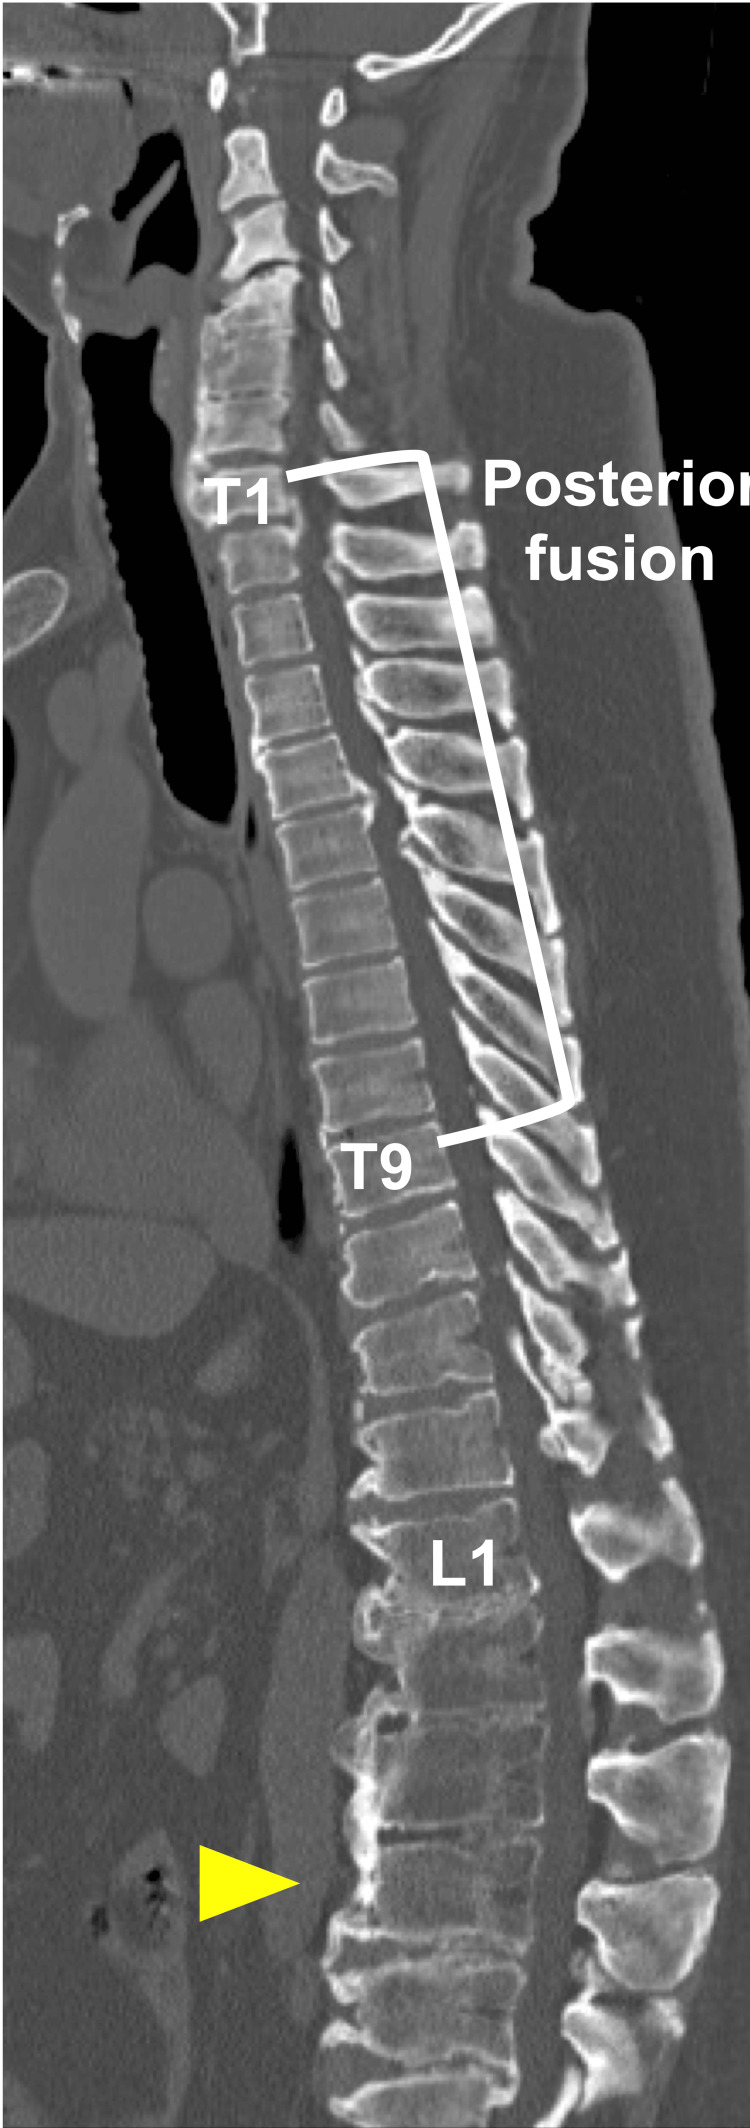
Preoperative whole spine sagittal reconstruction CT image There was a spontaneous fusion of the anterior longitudinal ligament ossification between L1 and S1 (yellow arrowhead). Additionally, the first surgery added posterior instrumented fusion from T1 to T9, limiting the intervertebral mobility of the thoracolumbar spine to the thoracolumbar junction only. CT: computed tomography
